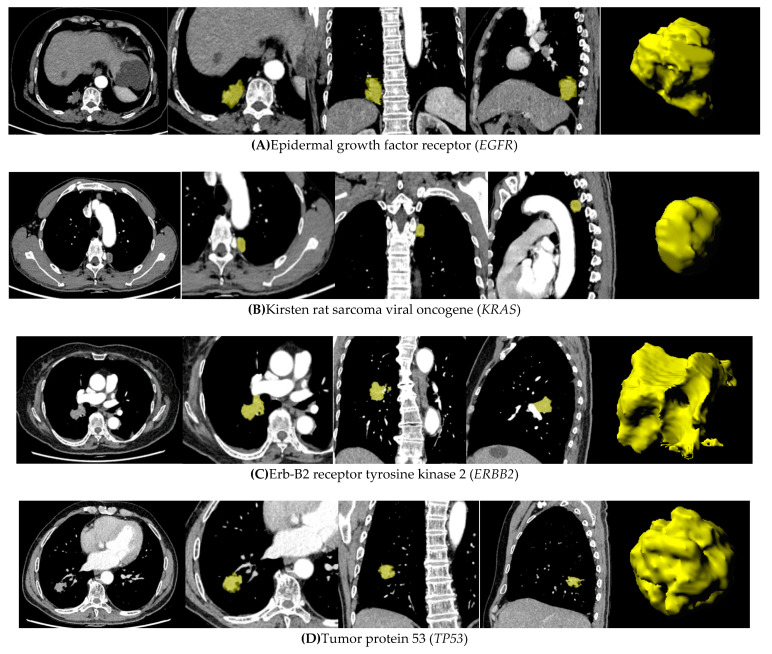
Representative CT images with tumor segmentation by a radiomics analysis platform. (A) A 30-year-old female non-smoker, with *EGFR* mutation in lung adenocarcinoma. A lobulated solid mass is observed in the lower lobe of the right lung. The maximum diameter is 23 mm. (B) A 64-year-old male smoker, with *KRAS* mutation in lung adenocarcinoma. A solid mass with rough margin is observed in the upper lobe of the left lung. The maximum diameter is 10 mm. (C) A 74 year-old female non-smoker, with *ERBB2* mutation in lung adenocarcinoma. A lobulated solid mass is observed in the middle lobe of the right lung. The maximum diameter is 18 mm. (D) A 66-year-old male smoker, with *TP53* mutation in lung adenocarcinoma. A lobulated solid mass with rough margin is observed in the lower lobe of the right lung. The maximum diameter is 12 mm.
